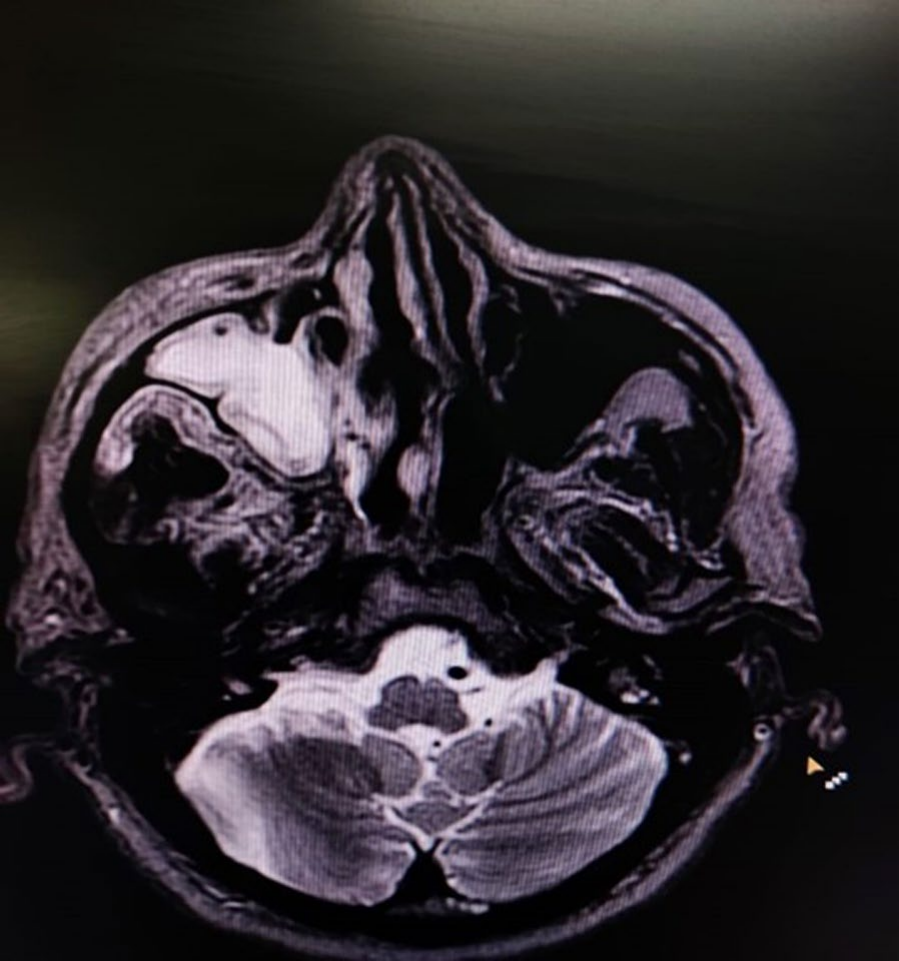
Contrast-enhanced MRI scan of nose and paranasal sinuses of a patient showing opacification of right maxillary sinus
